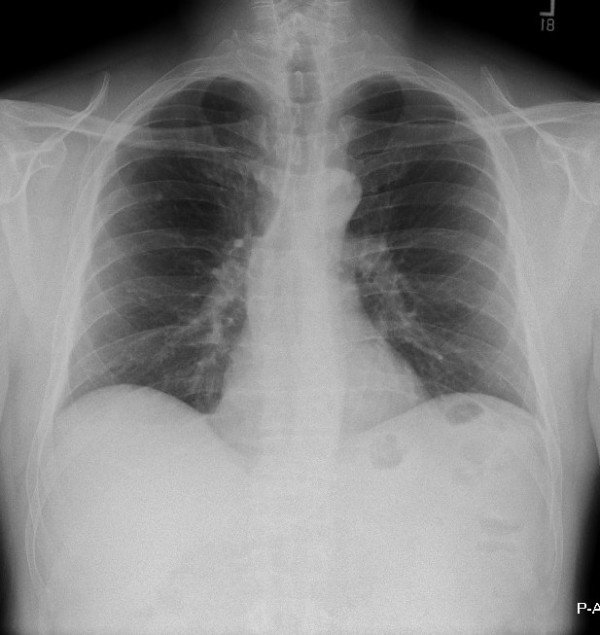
PA chest radiograph for patient #2. This film was interpreted as normal.
